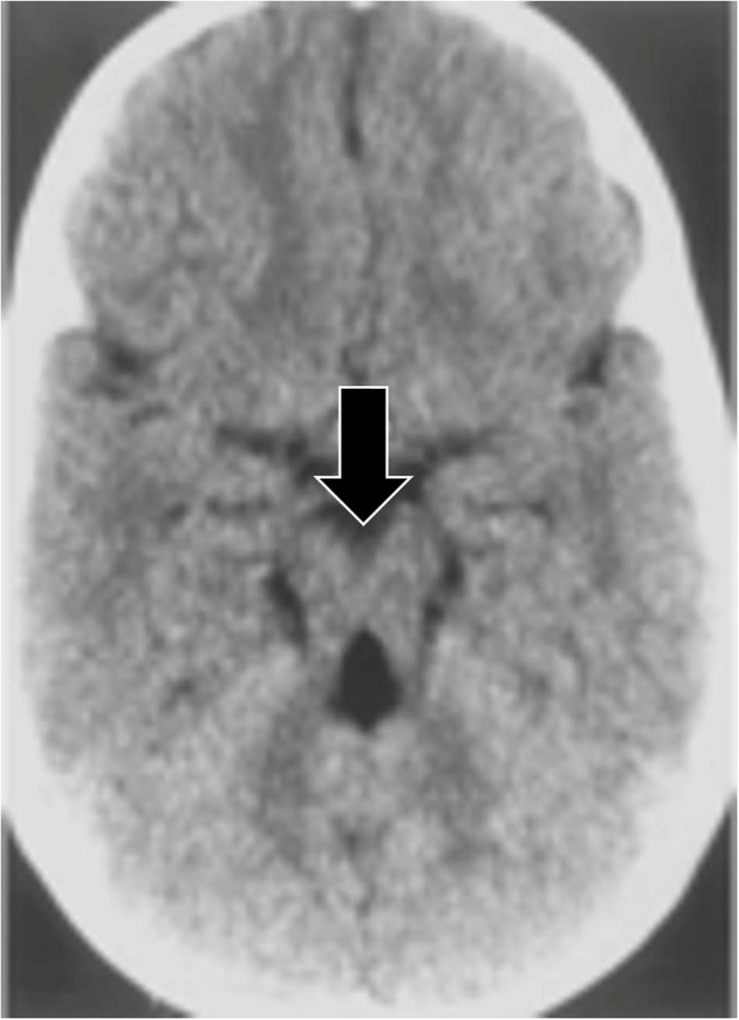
An axial CT image demonstrating the molar tooth sign, a pattern associated with Joubert syndrome. Lack of normal decussation of the fiber tracts of the superior cerebellar peduncles and the pyramids results in thickened and horizontally oriented superior cerebellar peduncles (McGraw, 2003; Romani et al., 2013). Along with the decreased anteroposterior dimension of the brainstem resulting from the absence of crossing fibers, and a deeper interpeduncular fossa (McGraw, 2003), these lead to the classic molar tooth appearance (from Gleeson et al., 2004).
